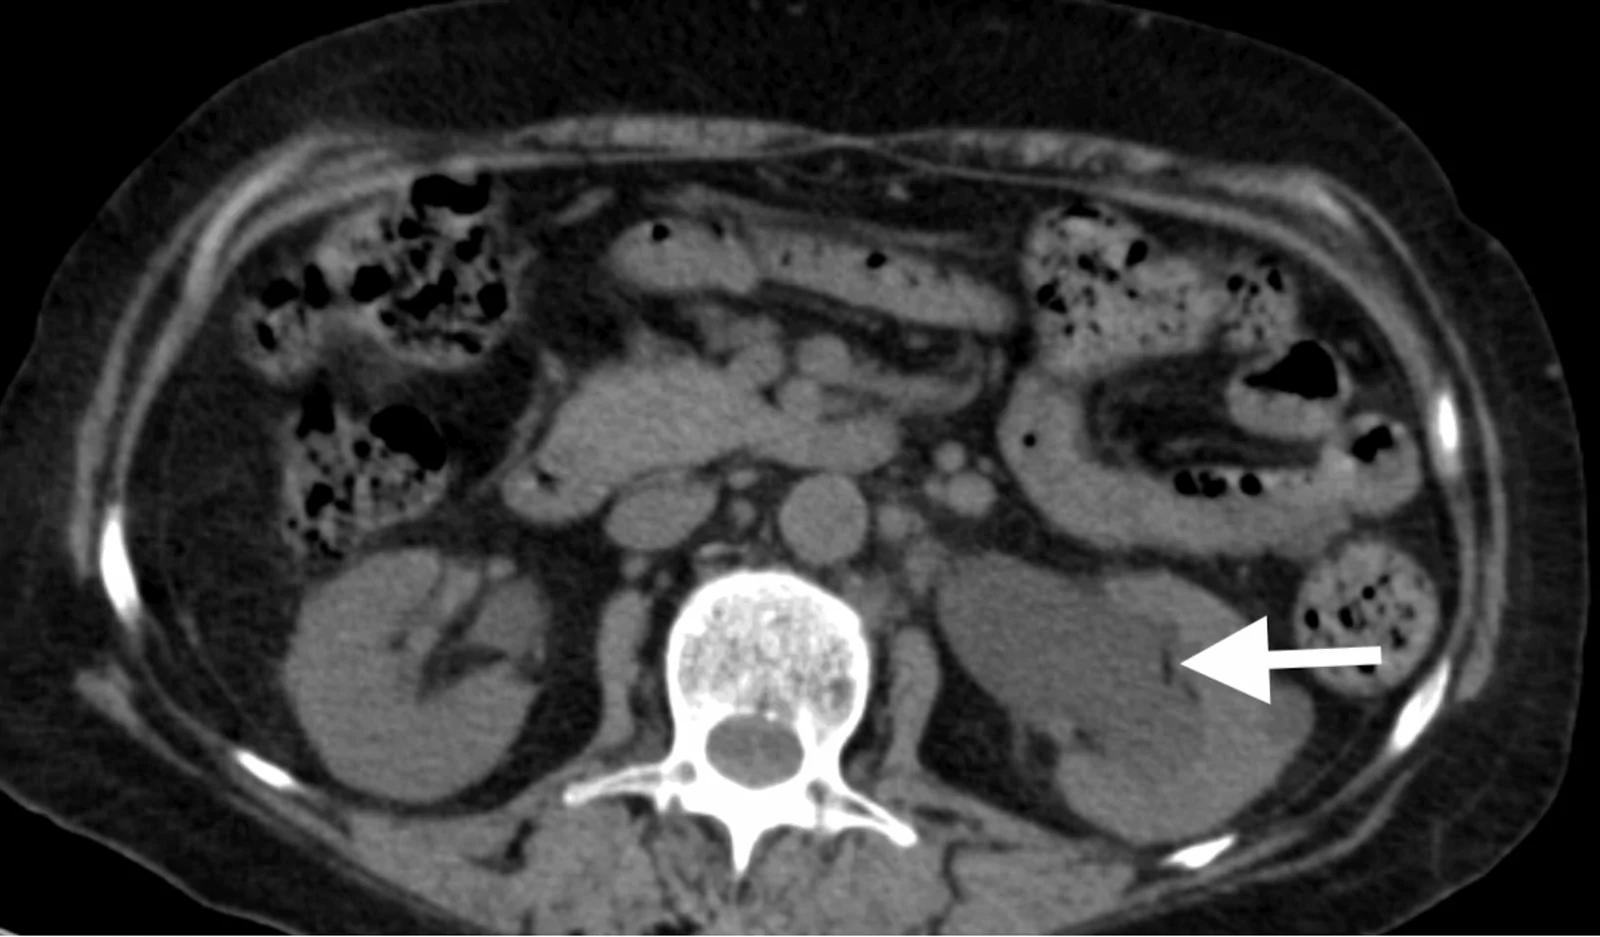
Axial non-contrast CT image of the abdomen showing left hydronephrosis (arrow). The image shows a grossly dilated left renal pelvis, as indicated by the arrow. The renal parenchyma appears normal in morphology and attenuation.
